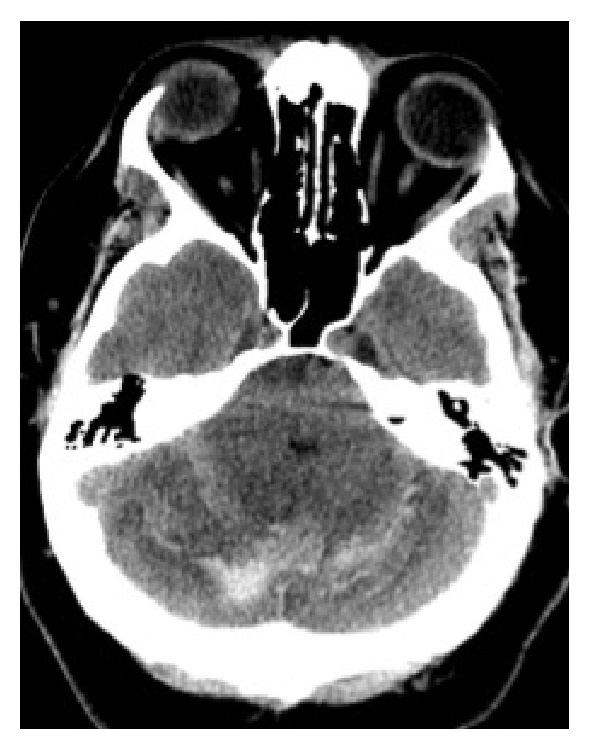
Nonenhanced CT head image at the level of the cerebellum demonstrates acute haemorrhages in both cerebellar hemispheres (arrow) with edema, causing mass effect on the fourth ventricle.
